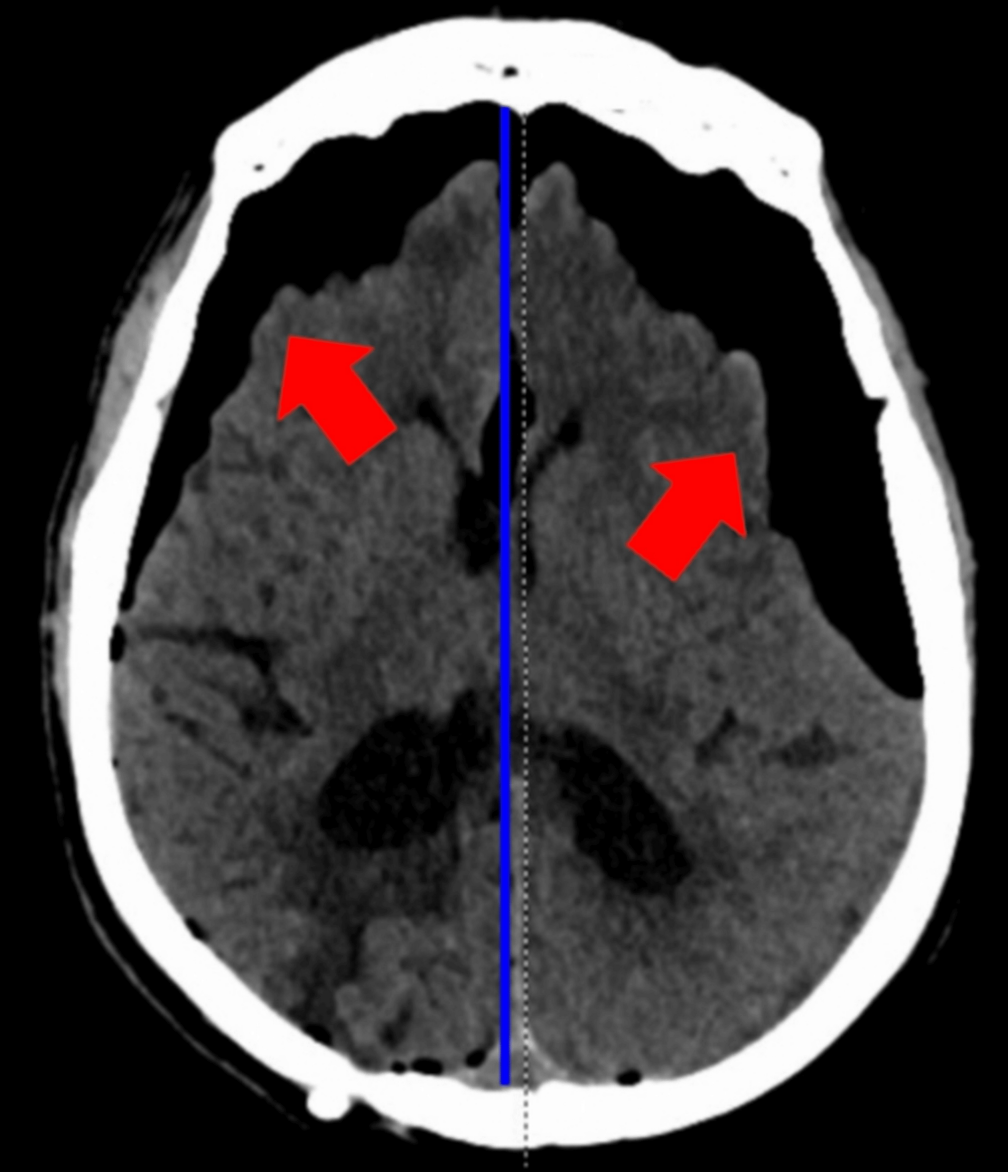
Non-contrast CT brain before neurosurgical intervention, depicting severe frontal pneumocephalus (left greater than right), with air extension in the skull and right parietal encephalomalacia, indicated by the red arrows. There is also a 4 mm rightward midline shift, with the midline depicted by the dashed line and the shifted midline depicted by the blue line.
